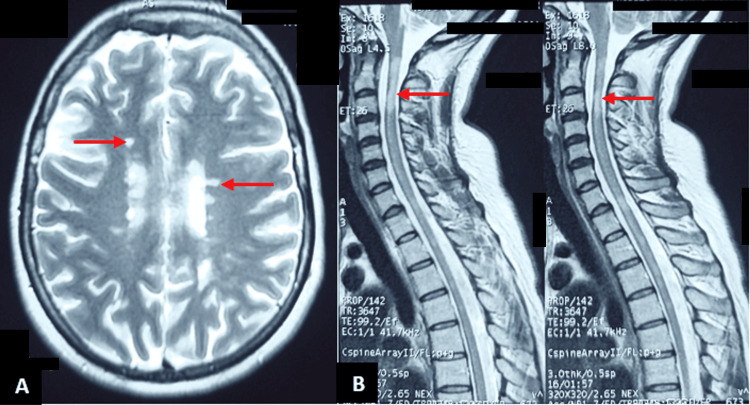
Brain and spinal abnormalities found in the second case presenting with rapidly progressive bilateral visual acuity deficit (VAD) associated with left hemifacial neuralgia (A) Brain MRI showing periventricular hyperintensities on T2. (B) Spinal cord MRI showing both longitudinally extensive spinal cord lesions over three vertebral segments and segmented ones.
